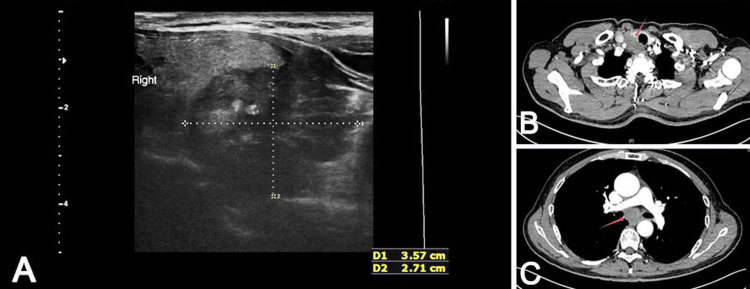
Imaging findings. (A) Neck ultrasound revealed a solid hypoechoic lesion (3.57 × 2.71 × 2.6 cm) in the right thyroid lobe. (B) CT demonstrated a soft tissue mass at the right thoracic inlet involving the right thyroid and trachea. (C) CT showed an irregularly shaped mass in the mid-to-distal esophagus.
